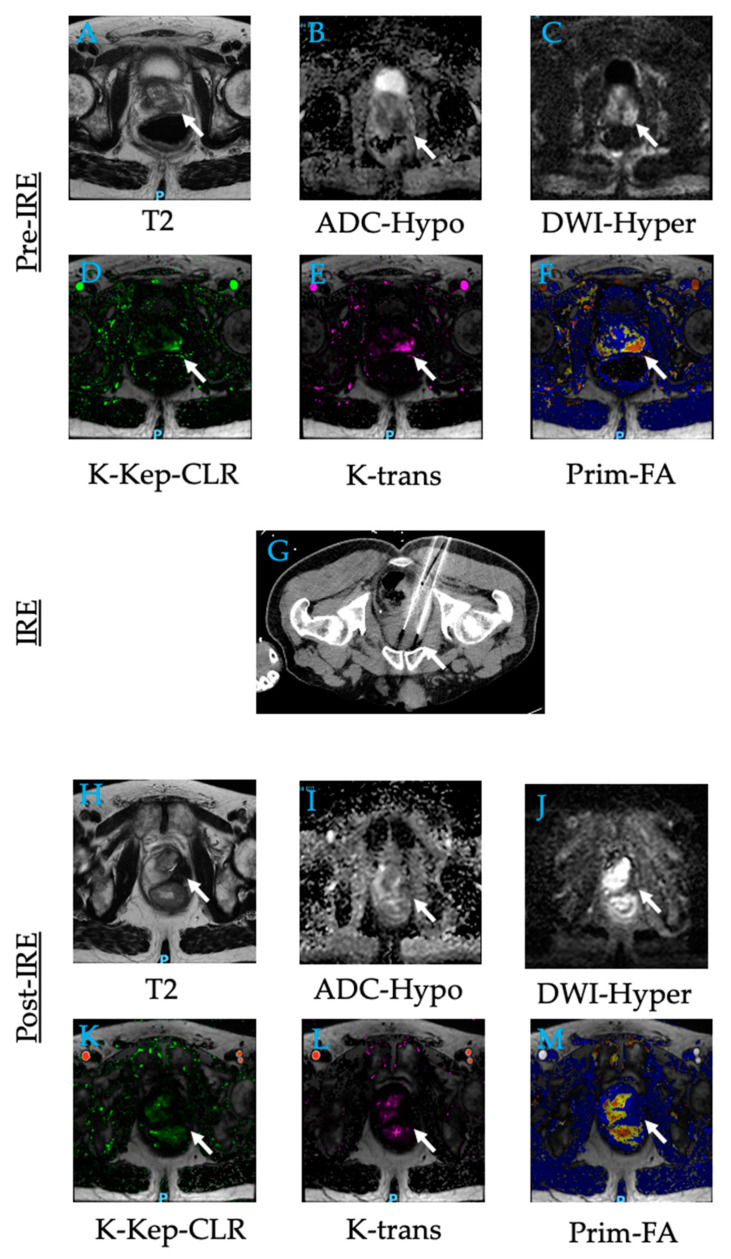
Irreversible electroporation of left prostate adenocarcinoma. A 64-year-old male with elevated PSA for which he underwent biopsy that showed adenocarcinoma in the left base of the prostate (white arrow) with Gleason score 7 and 8. (A–F) Contrast MR image of the prostate demonstrated a mass in the left peripheral zone. (G) He underwent IRE for the left side of his prostate. (H–M) Follow-up MR images show no residual tumor after 12 months. Abbreviations: ADC, apparent diffusion coefficient color-coded perfusion maps; DWI, diffusion-weighted imaging; DCE, color-coded dynamic contrast enhancement perfusion maps including K-Kep-CLR, K-trans, and Prim-FA.
